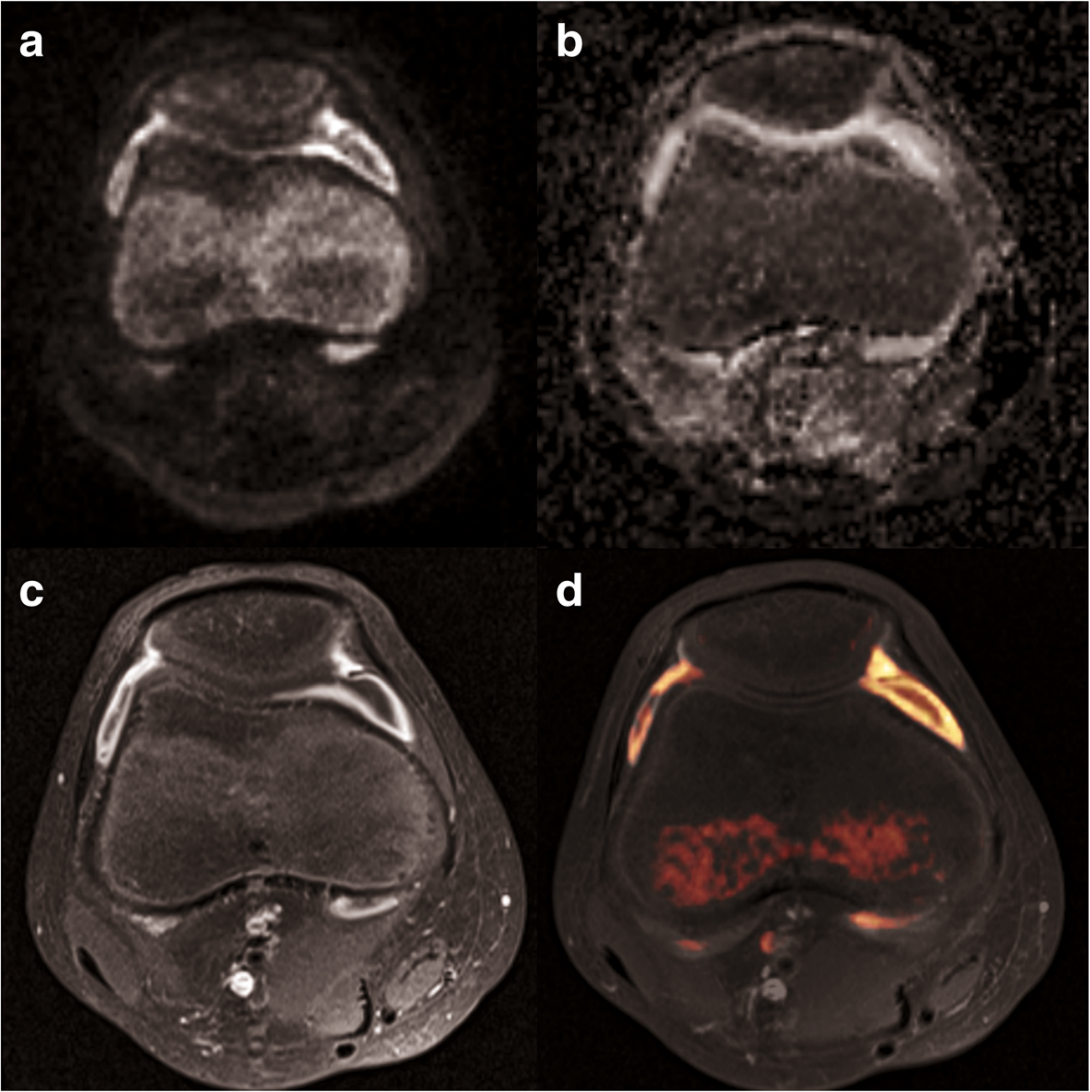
Twelve-year-old male JIA patient, persisting clinical symptoms with methotrexate treatment. DWI b = 800 s/mm^2^ (a), ADC map (b), contrast-enhanced MRI (c) and image fusion (d) of colorised overlay of DWI signal onto image (c) with 3D Fusion software (Siemens Medical) all show active synovitis and small amounts of joint effusion in the anterior and posterior joint space. Image acquisition with multi-shot read-out segmented DWI (RESOLVE, Siemens) is virtually free of artifacts
